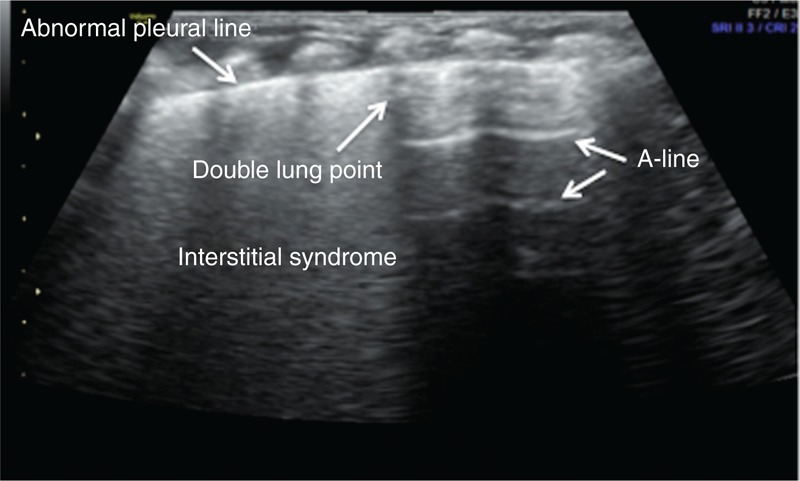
Ultrasonic manifestation of TTN: partial A-line disappearance. The infant above had a GA of 31 wk and was delivered vaginally; the infant had a birth weight of 1550 g. The infant was admitted to the hospital 5 h after birth after suffering from dyspnea for 5 h. Lung ultrasonography showed clear double-lung point, interstitial lung syndrome, and disappearance of the A-line at the interstitial lung syndrome site (there were A-lines at other sites). GA = gestational age, TTN = transient tachypnea of the newborn.
